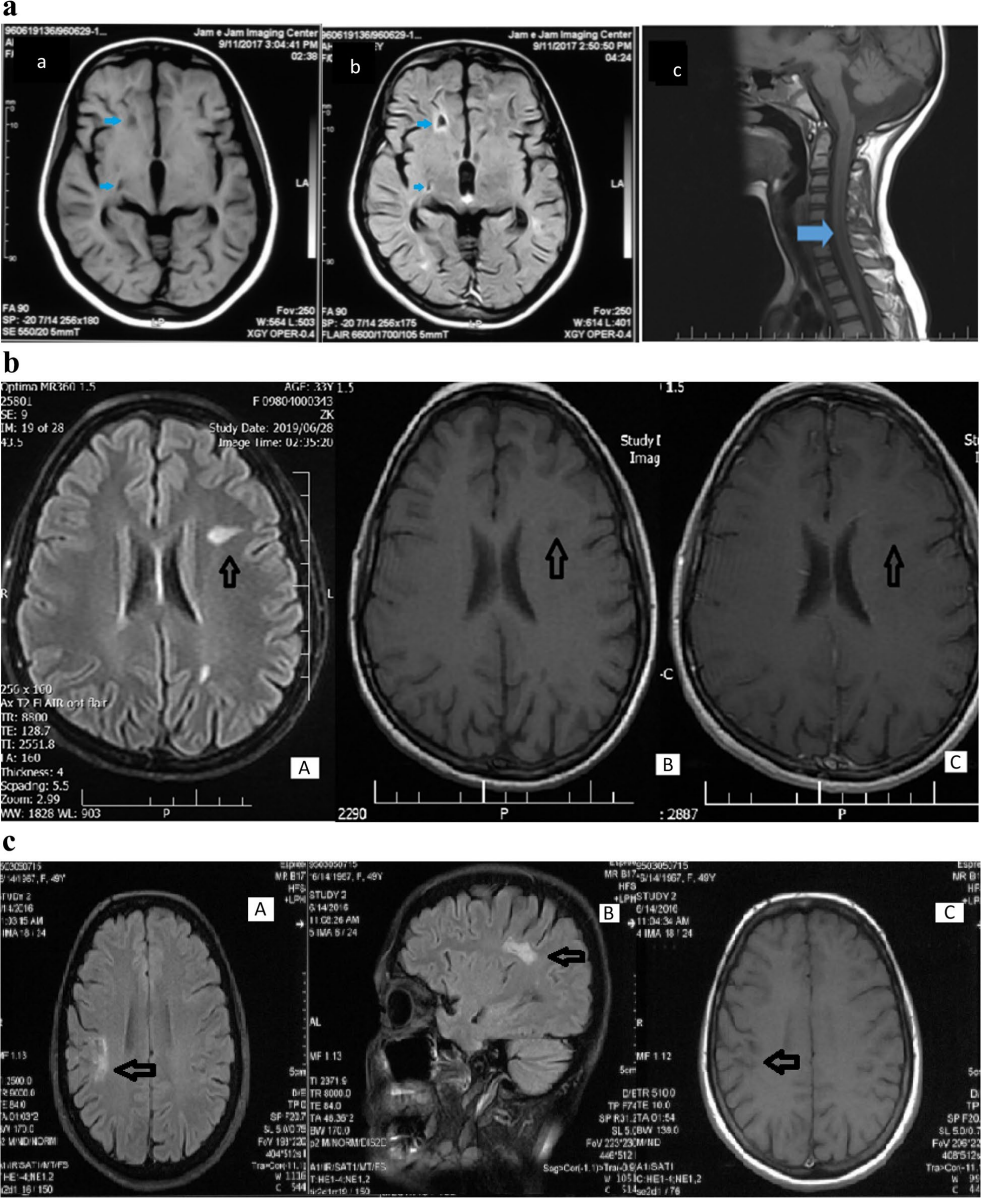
A Example of T1 Hypointense lesions in a 23-year-old female with NMOSD and positive AQP-4 test. The visible T1 hypointense lesions are depicted in the white matter on T1 (a) and corresponding FLAIR(b) images located in the hemispheric and periventricular white matter. No enhancement was noted in T1 enhanced images (not shown in the Figure). Also, the centrally-located long T1 hypointense lesion is shown in cervical cord (c). B Hemispheric white matter T1 hypointense lesions (black arrows) in a 33-year-old female; The most prevalent location of T1 hypointense lesions in chronic phases of Neuromyelitis Optica. A FLAIR axial sequence. B T1-weighted axial sequence. C T1-weighted post contrast sequence; No obvious enhancement after contrast injection appears in the lesion. C Hemispheric white matter T1 hypointense lesion (black arrows); A FLAIR axial sequence. B FLAIR sagittal sequence. C T1-weighted axial sequence
